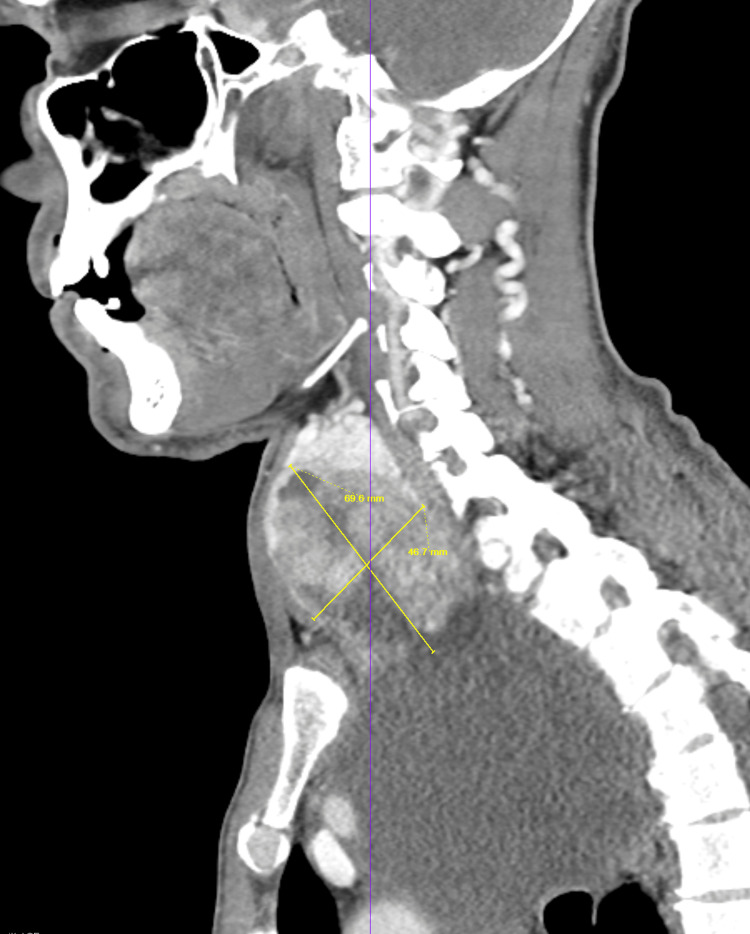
CT neck and chest showing a large right thyroid mass CT scan of the neck and chest demonstrating a large right thyroid mass measuring approximately 7.5 cm with features suspicious for malignancy. CT, computed tomography.
